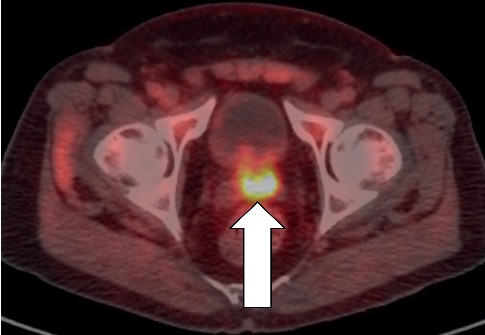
Image of a 72-year-old male who had undergone Calypso-guided intensity-modulated radiotherapy and cyberknife treatment of a right-sided iliac lymph node (identified on a prior ^18^F-fluciclovine study). He was referred for ^18^F-fluciclovine imaging with PSA rising to 15.1 ng/mL. This axial, fused PET/CT image shows intense ^18^F-fluciclovine uptake in the left side of the prostate gland (white arrow), consistent with prostate cancer recurrence.
